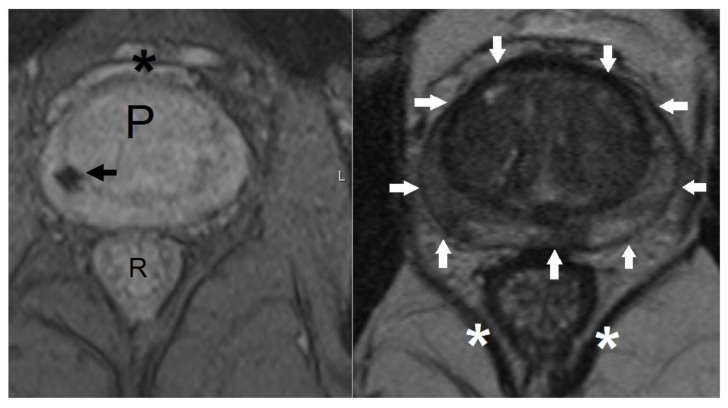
T2-weighted magnetic resonance (MR) sequences used for fusion with computerised tomography (CT) planning scan. Axial gradient echo (left pane) and T2 fast spin echo (right pane) MR images at the level of the prostate. Left pane: low signal void (black arrow) represents the site of a fiducial marker. P, prostate; R, rectum; *, venous plexus of Santorini. Right pane: white arrows show position of prostatic capsule. *, levator ani muscles.
